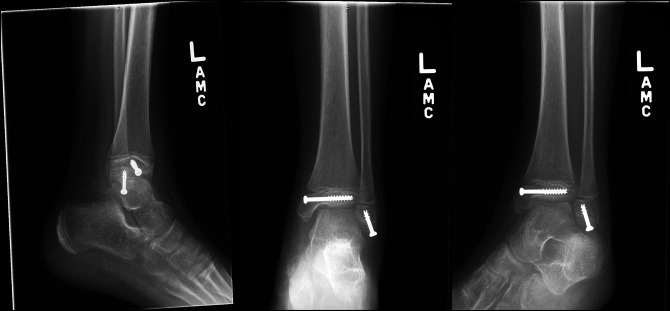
Postoperative AP, mortise, and lateral view ankle radiographs after surgical fixation.
